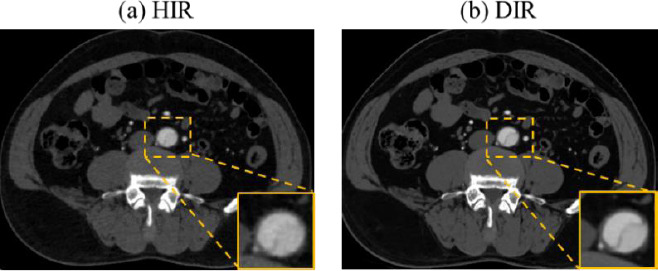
Aortic CTA images obtained in a 49-year-old man with aortic dissection. Images were acquired at a CTDI_vol_ of 8.85mGy and reconstructed with HIR (a) and DIR (b). The boundary between true lumen and false lumen is more clearly delineated by DIR, at the distal segment involved with the dissection.
